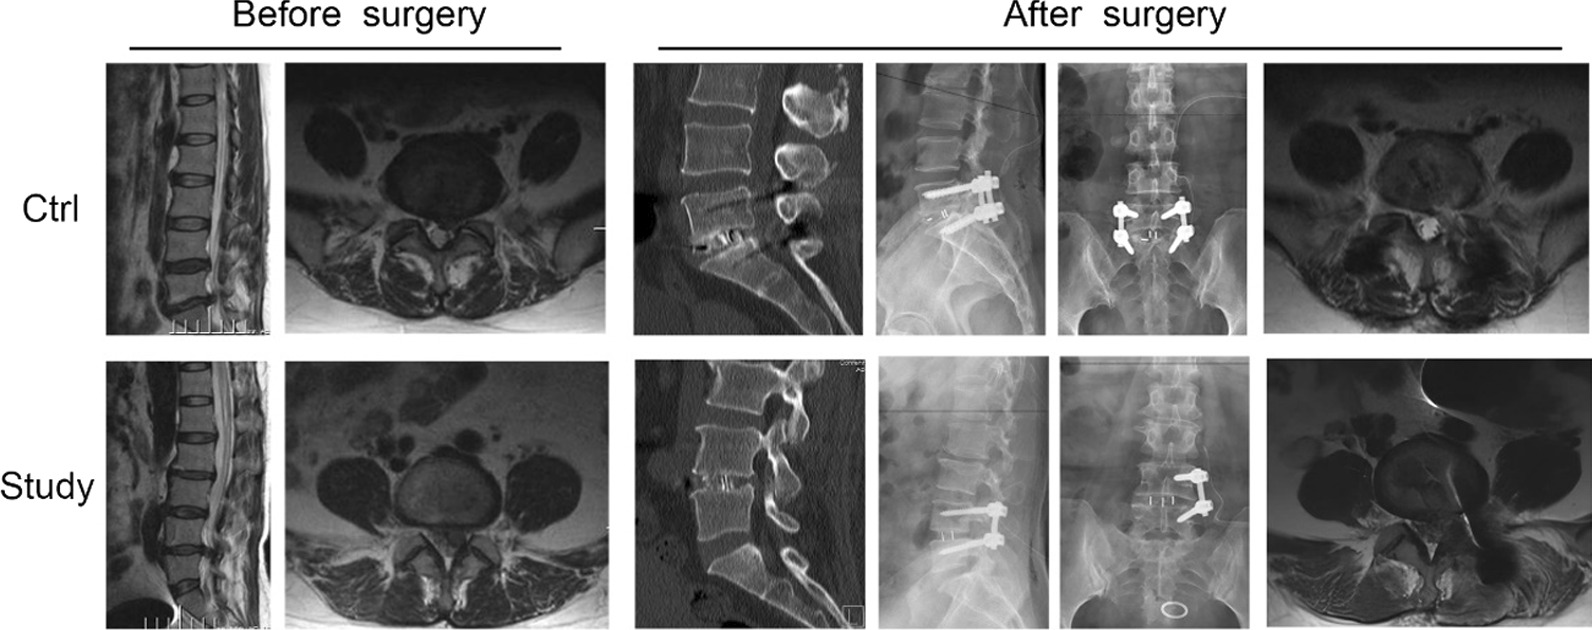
Images shown from left to right are MR sagittal; MR axial; CT sagittal; X-ray lateral; X-ray frontal and MR axial
